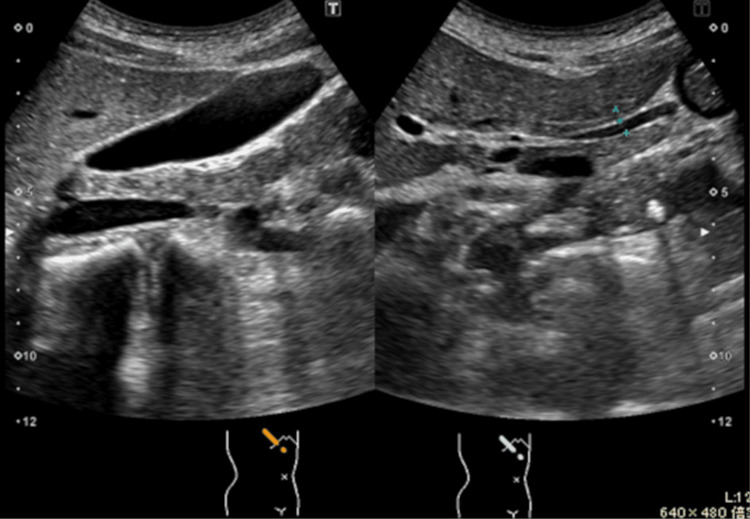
Abdominal ultrasound findings not suggestive of biliary disease
